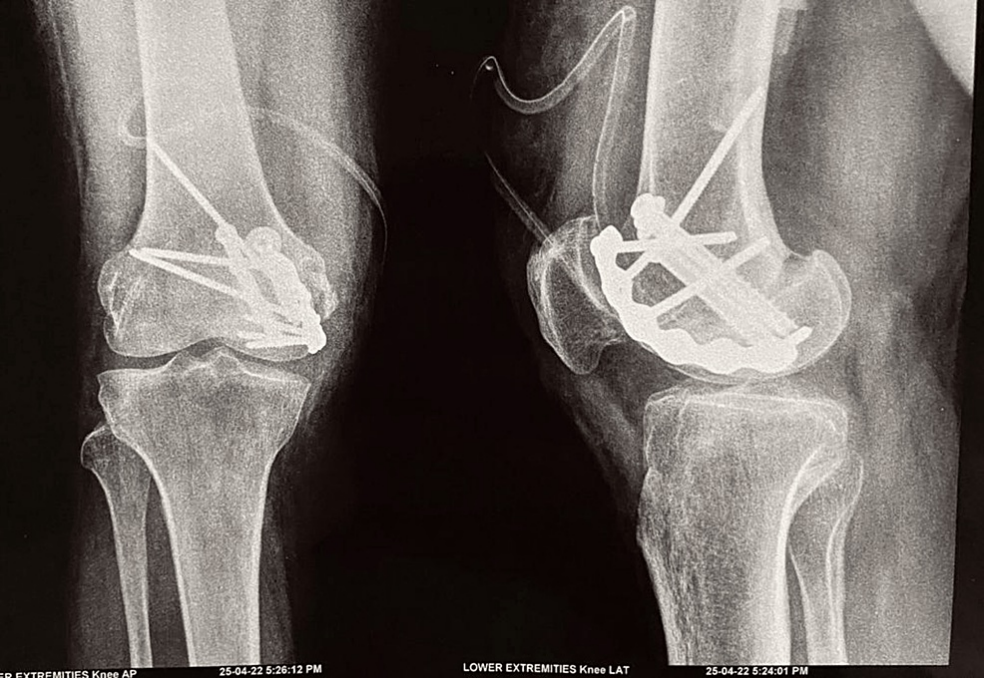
Post-operative plain radiograph of the right knee. Fracture fragment has been fixed using cancellous screws and reconstruction plate
